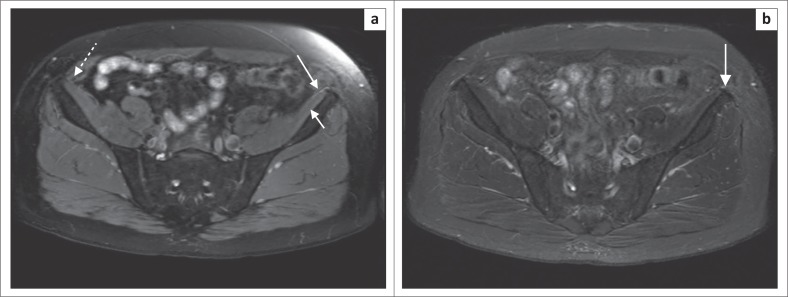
3T proton density fat-saturated axial MR images at the level of the anterior superior iliac spine in a 54-year male patient presenting with a clinical suspicion of meralgia paresthetica on the left. Note the high signal and prominence of the lateral femoral cutaneous nerve on the left (long arrow) as compared to the right (dashed arrow) as it runs over the (a) iliacus muscle (short arrow) and (b) anterior superior iliac spine.
